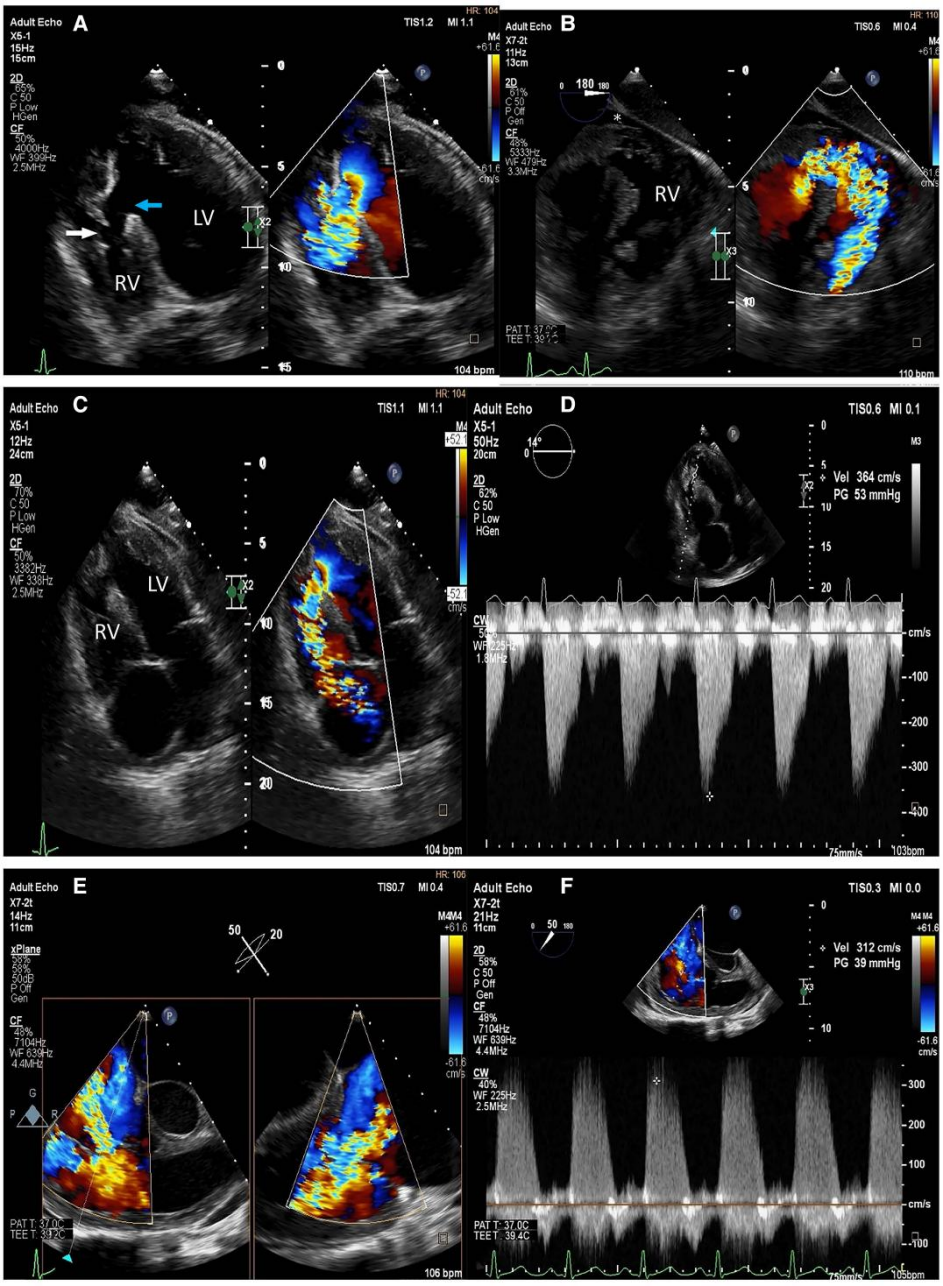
The transthoracic echocardiography (*A*), off-axis four-chamber(4C) view, shows (blue arrow) a large iatrogenic muscular ventricular septal defect (VSD) that measured 2 cm in size with a left to right shunt (LV, left ventricle; RV, right ventricle). There was also disruption of the moderator band (white arrow). (*B*) Shows the transoesophageal echocardiography transgastric view with a large serpentine jet extending form the defect and merging with the functional tricuspid regurgitation (TR) jet and a small pericardial effusion (marked with an asterisk). This can also be noted in the apical 4C colour compare images (*C*). (*D*) depicts the continuous wave (CW) Doppler with angle correction across the VSD and a peak gradient of 53 mmHg consistent with a large defect. (*E and F*) focuses on the TR jet with a right ventricular systolic pressure of 39 mmHg due to left to right shunting of blood.
